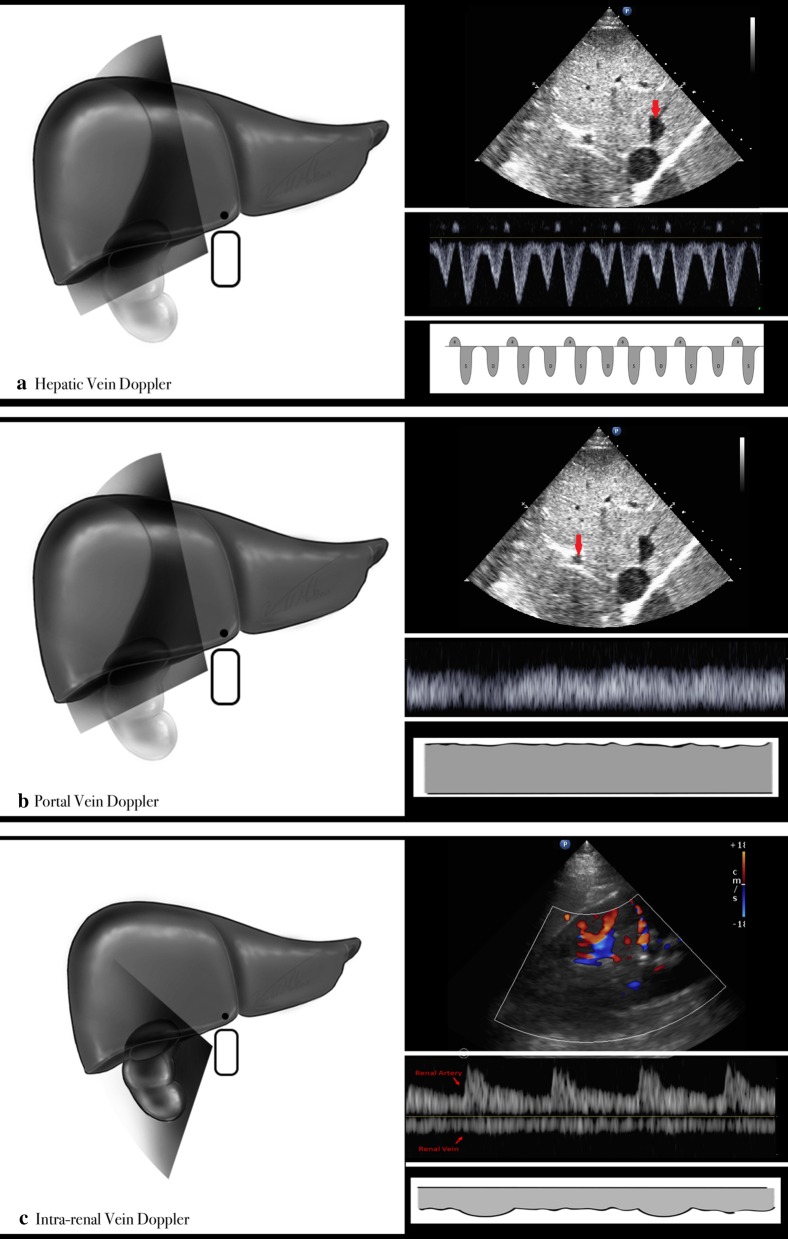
Ultrasound surface and B-mode anatomy and normal venous Doppler waveforms
